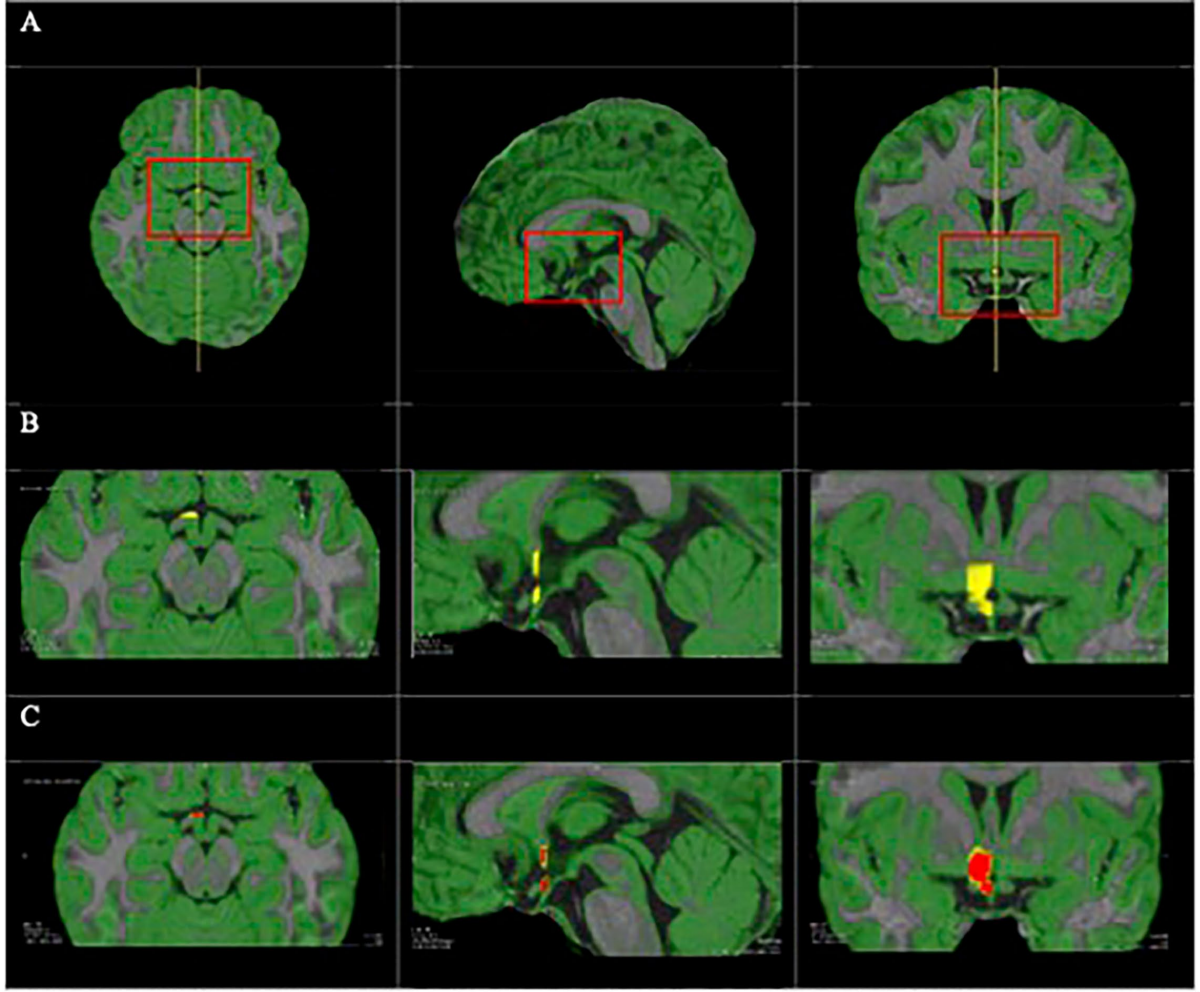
(A) Triplanar view with T1-weighted image and GM reference as a green overlay; full view (left) and zoomed in on the hypothalamus (right). Volumetry of preoptic hypothalamus: ROI in yellow (B) and seed growing step in red (C).
